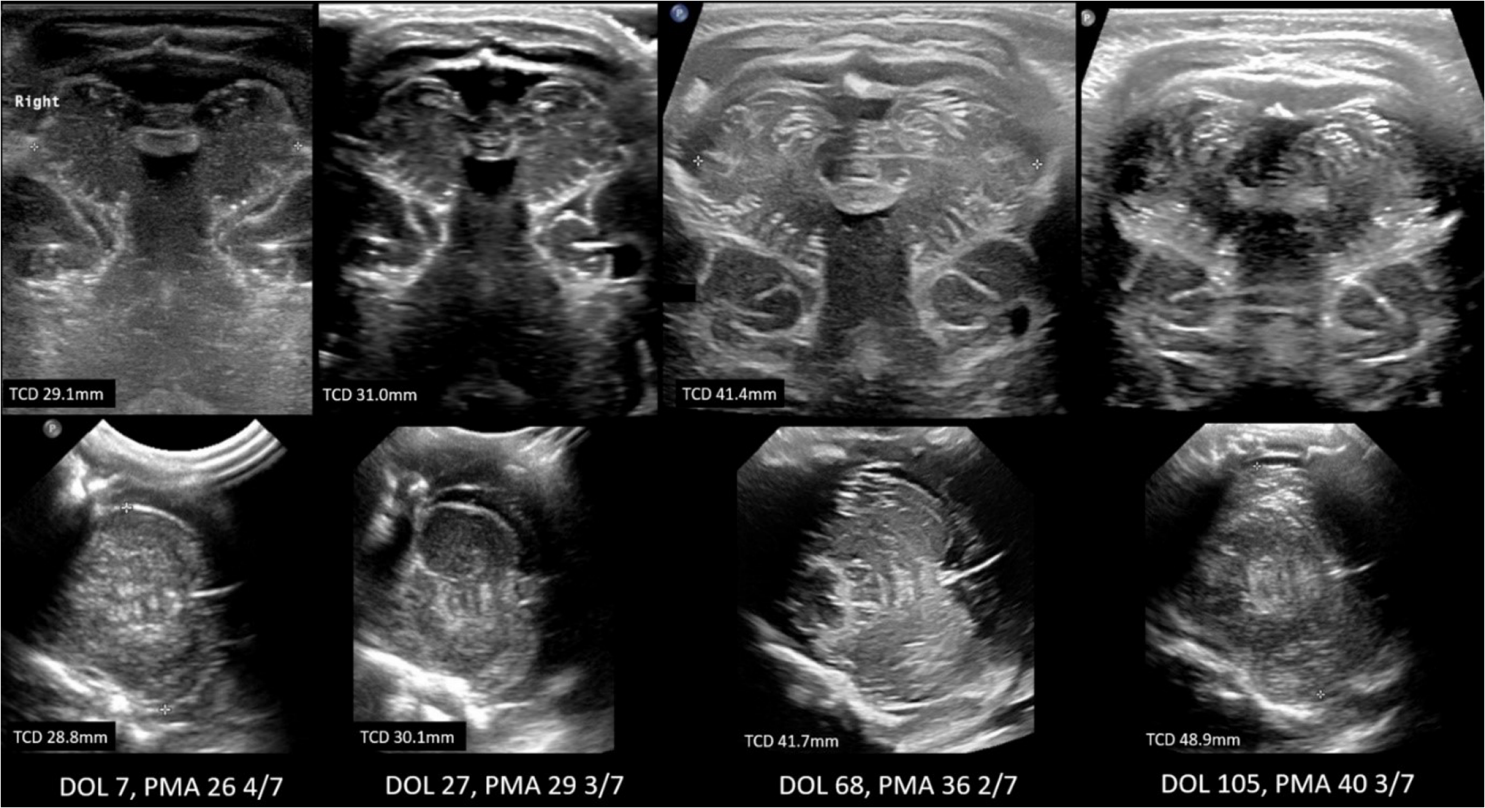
Cerebellar hypoplasia of prematurity: Preterm infant of 25 4/7 weeks gestation age without supra- or infratentorial hemorrhage. Upper row shows serial transnuchal ultrasound scans, the lower row corresponding axial scans through the mastoid fontanel at day of life (DOL) 7 and 27, approximately postmenstrual age (PMA) 36 weeks and term equivalent age (TEA). A normal transverse cerebellar diameter (TCD) of 25-50th percentile according to Imamoglu et al. [29] was present at first scan with one week. Even without recognizable supra- or infratentorial hemorrhage in serial ultrasound scans a mild cerebellar hypoplasia developed during the unremarkable neonatal course with a TCD < 3rd percentile at PMA 36 weeks and TEA
